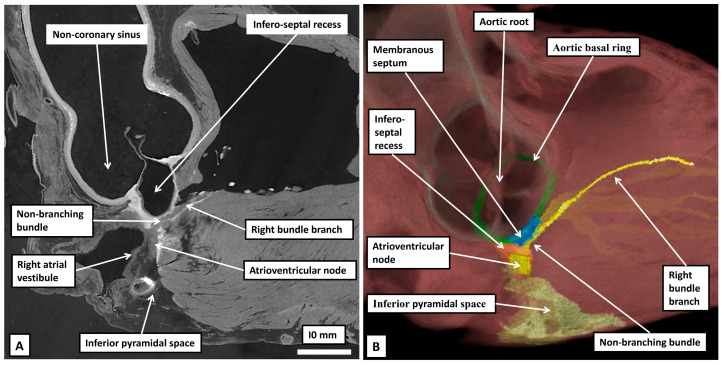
The images are taken from a human heart scanned using HiP-CT technology at the European Synchrotron Radiation Facility, made available at 19.89 um, in this case, of the heart of the body donor s-20-29 [3]. (A) Panel A is a two-dimensional image, showing the transition from the atrioventricular node at the apex of the inferior pyramidal space, through the non-branching bundle located within the infero-septal recess, and to the continuation as the right bundle branch. (B) Panel B then shows how it is possible to reconstruct these features within the heart itself, showing the relationship of the ventricular components of the axis to the virtual basal ring of the aortic root.
